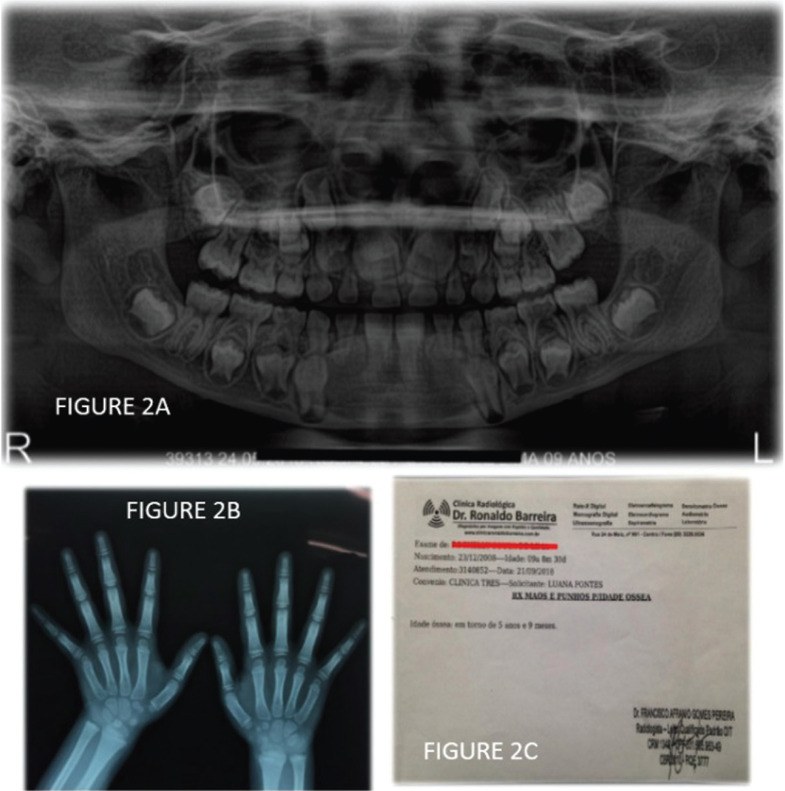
A) Panoramic radiograph of the patient. B) Radiograph of the patient’s hand and wrists. C) Hand and wrist radiography report showing that the bone age corresponds to 5 years and nine months.
